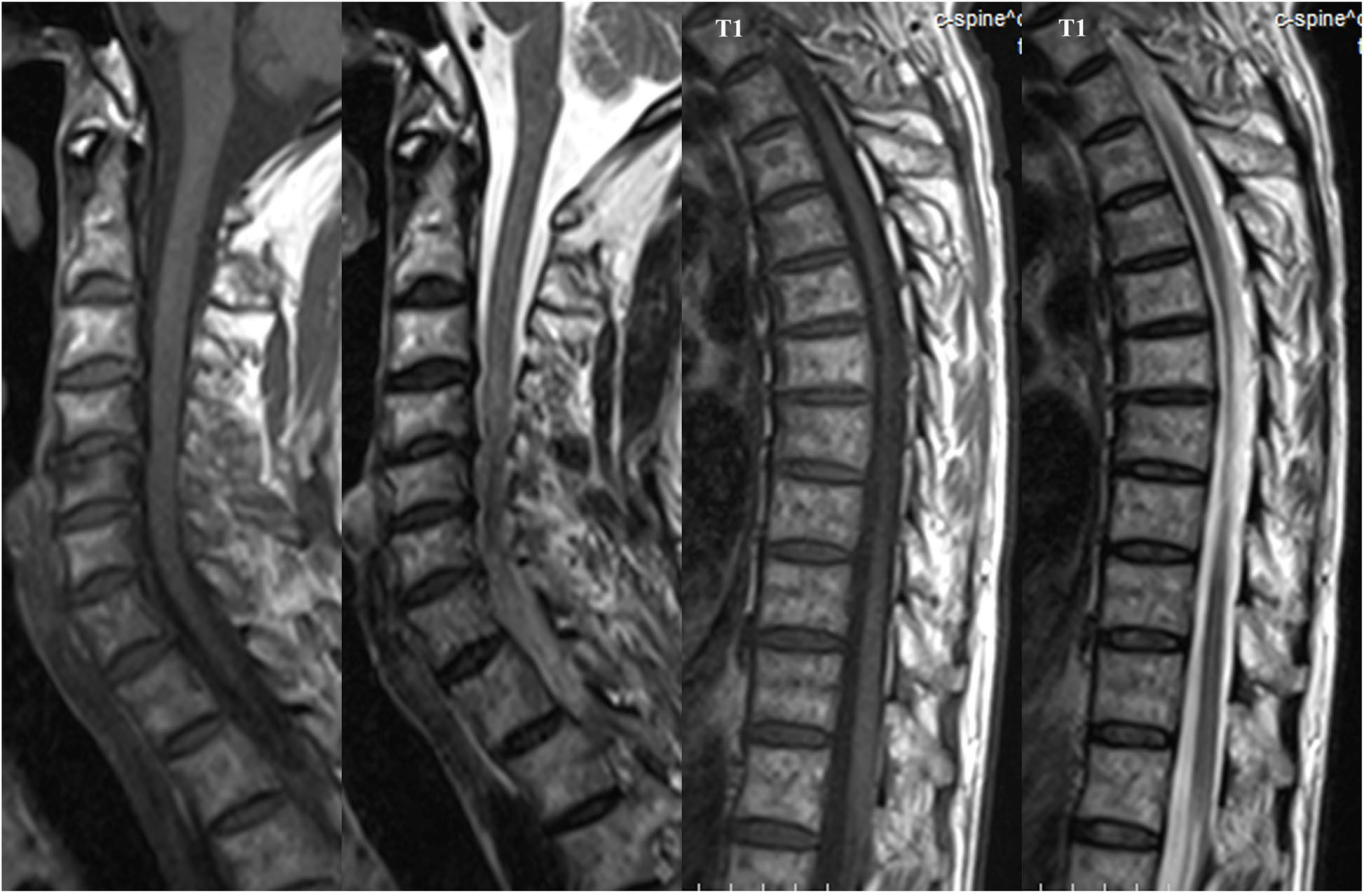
Spinal MRI did not demonstrate silicone oil migration into the subarachnoid space of the spinal cord
